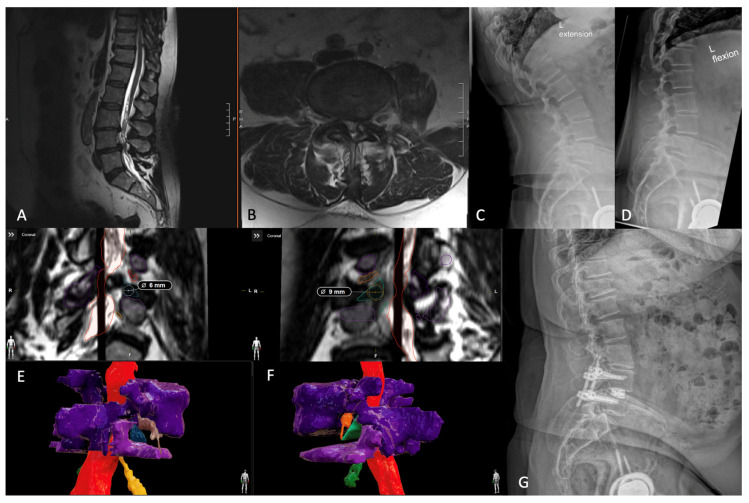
(A,B) Preoperative T2-SPACE-weighted 1 mm slice MRI showing L4-5 grade 1 spondylolisthesis on both sagittal and axial views. (C,D) Preoperative extension and flexion radiographs further depicting the instability of the pathologic L4-5 facet joints. (E) BrainLab’s planning software highlighting the left trans-Kambin corridor (outlined in blue) and (F) the right trans-Kambin corridor (outlined in green), with the maximum permissible cannula measurement overlaying each area and 3D reconstrued images below. (G) Postoperative radiograph confirming appropriate hardware placement and correction of their spondylolisthesis.
